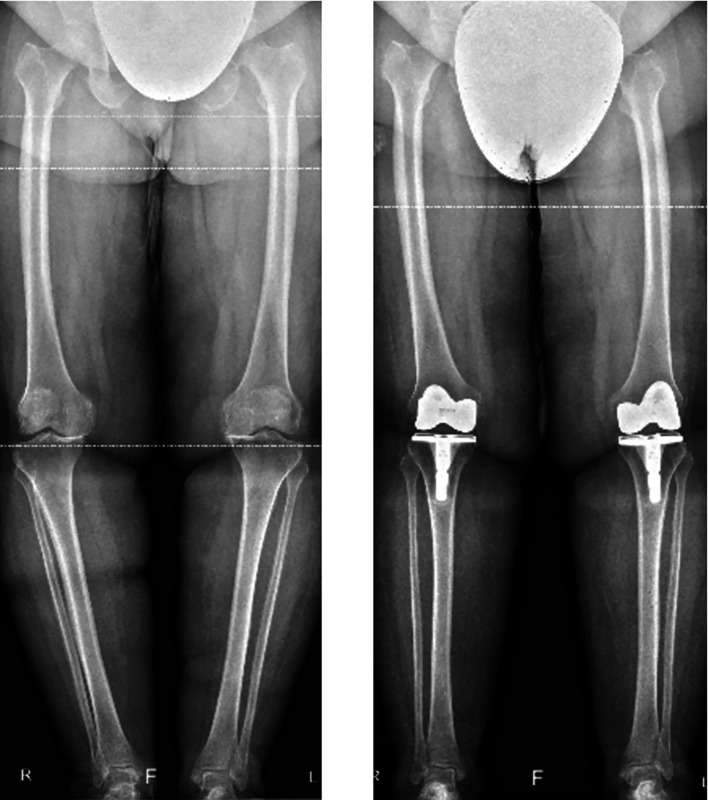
Preoperative and postoperative X-rays of a patient with a BMI of 42 who underwent bilateral total knee arthroplasty with a short tibial stem.
